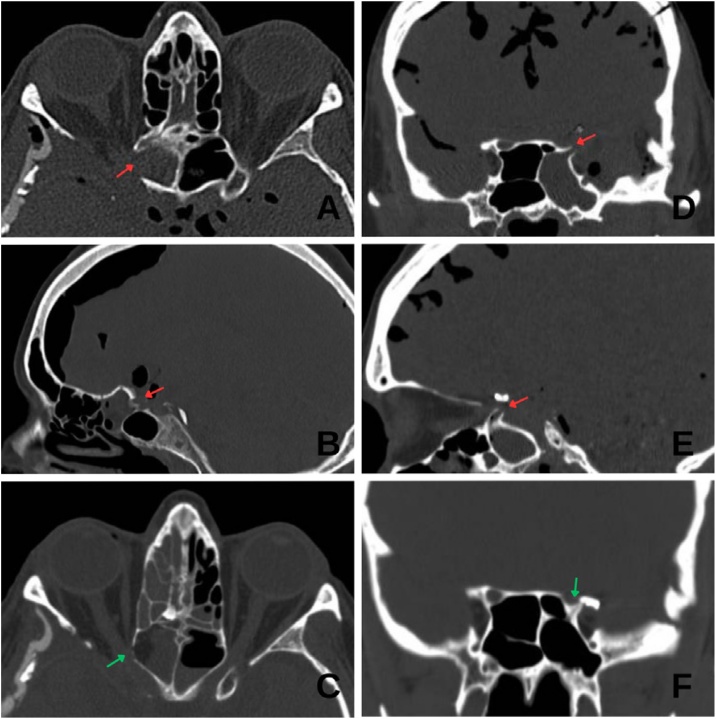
Pre- and postoperative CT scans of Cases 1 and 2. (A and B) Axial and sagittal preoperative views of Case 1 showing marked pneumocephalus and the defect area (red arrow). (C) Postoperative axial view of Case 1 showing resolution of the defect (green arrow). (D and E) Coronal and sagittal preoperative views of Case 2 showing pneumocephalus and the defect (red arrow). (F) Postoperative coronal view showing resolution of the defect and absence of pneumocephalus (green arrow).
